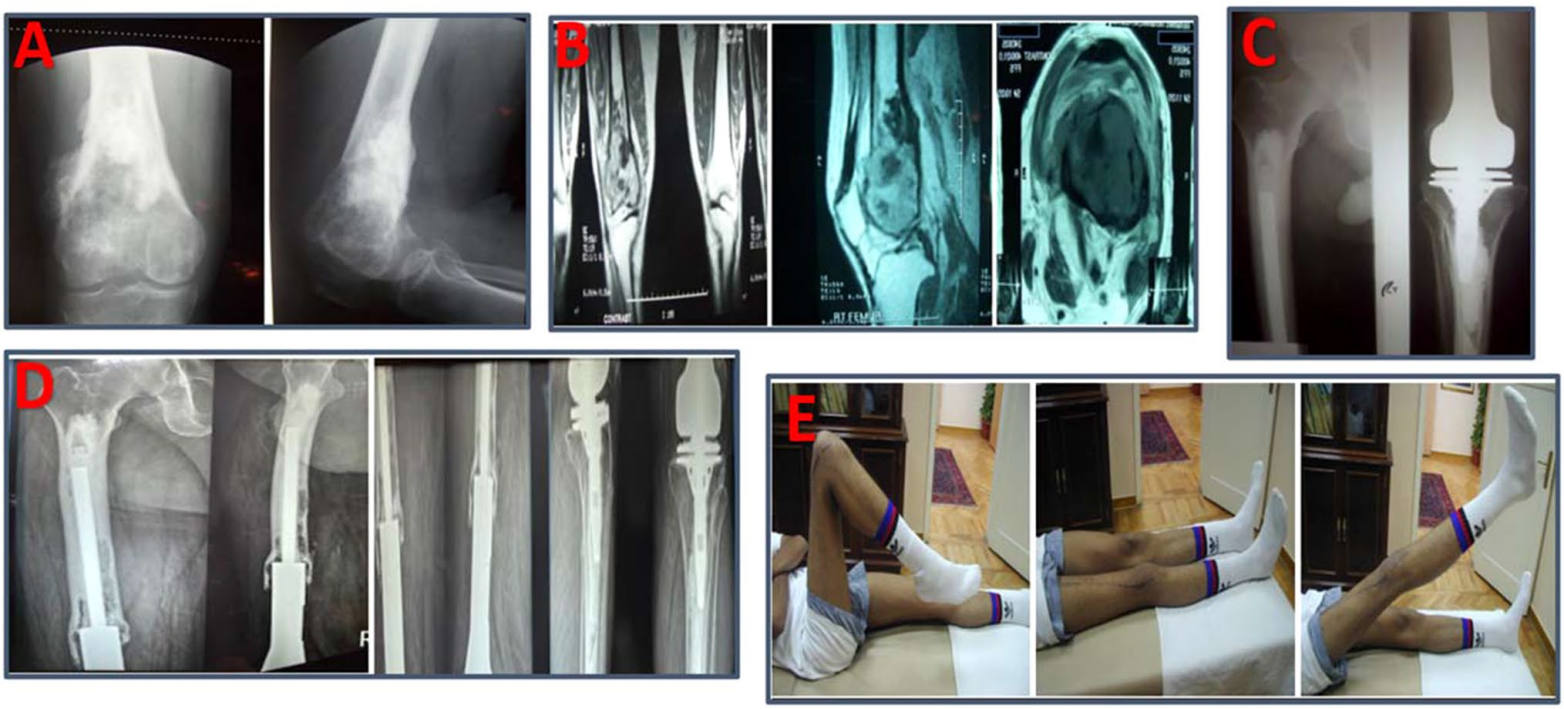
57 years of male with right distal femoral osteosarcoma and CT chest was free. A Pre-operative X-rays. B Pre-operative MRI. C Post-operative. D 11 years follow-up X-rays. E Final range of motion with MSTS score 30
